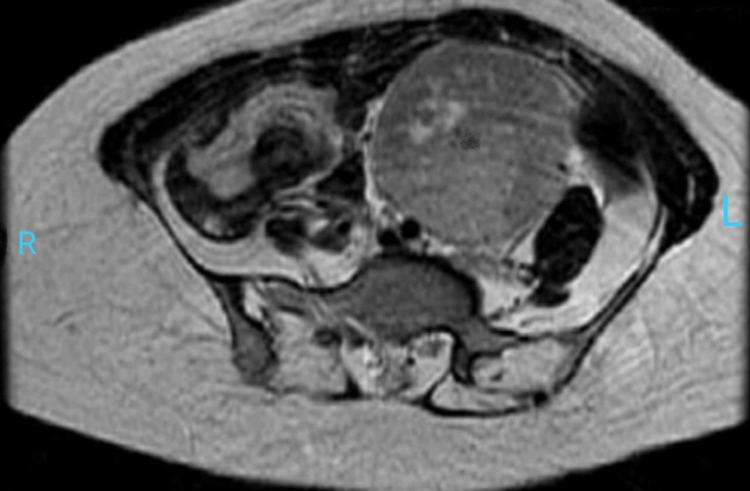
T2-Weighted MRI showing retro-peritoneal left-sided well-circumscribed, heterogeneous, and hyper-intense mass.
